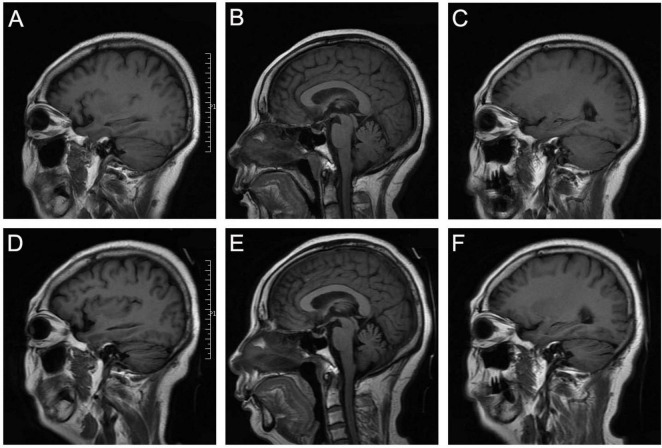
The radiology images. (A-C) Initial sagittal T1-weighted FLAIR sequences at baseline assessment. (D-F) Follow-up sagittal T1-weighted FLAIR sequences at terminal evaluation, demonstrating development of cerebellar atrophy.
